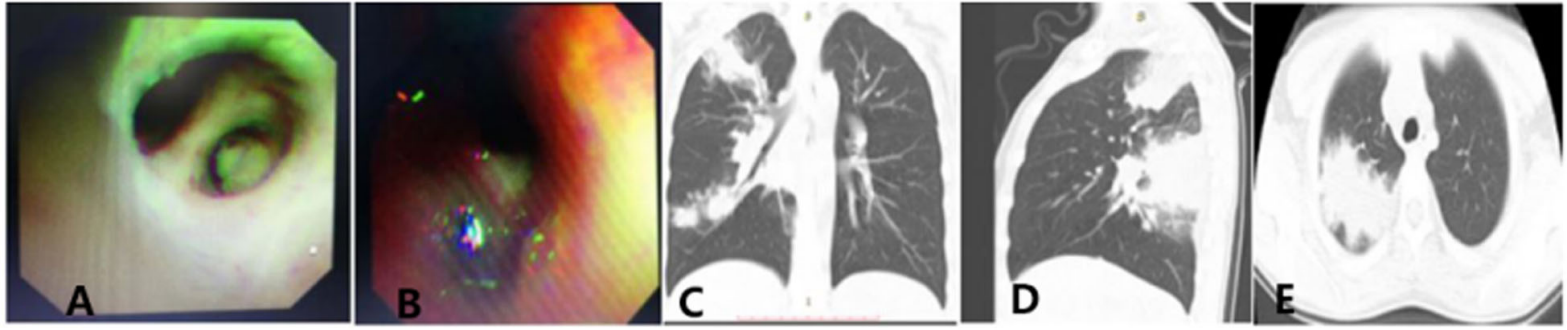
This is a bronchoscopic performance and imaging features of a MPP patient. Bronchoscopy performance (A and B): A is the plastic sputum plug of the upper right and the posterior branch. The wall of the tube is smooth after the brush removes it; B is the shaped sputum plug on the left side of B8 and B9, and the lumen is smooth after lavage; C and D are the normal and lateral pictures of this child’s chest radiograph; and E is a CT slice of this child. CT = computed tomography; MPP = *M. pneumoniae* pneumonia
